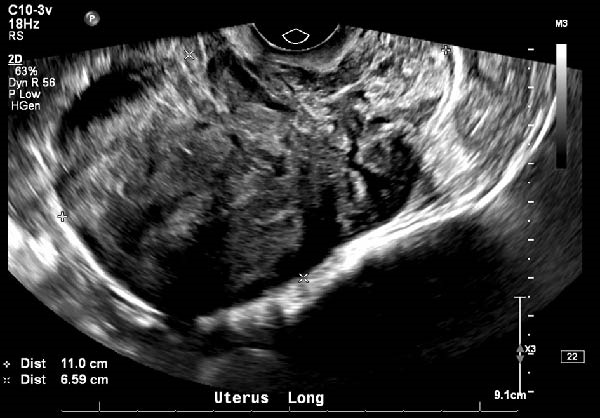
Transvaginal ultrasound image of leiomyoma.
